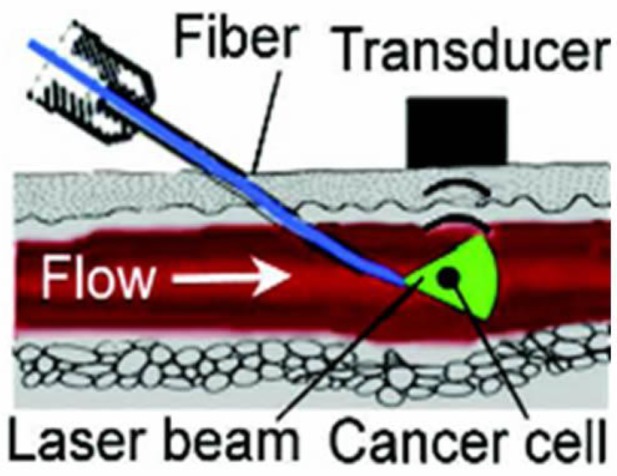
Minimally invasive fiber delivery of laser radiation into blood vessels with detection of acoustic waves by an ultrasound transducer on the skin surface.
